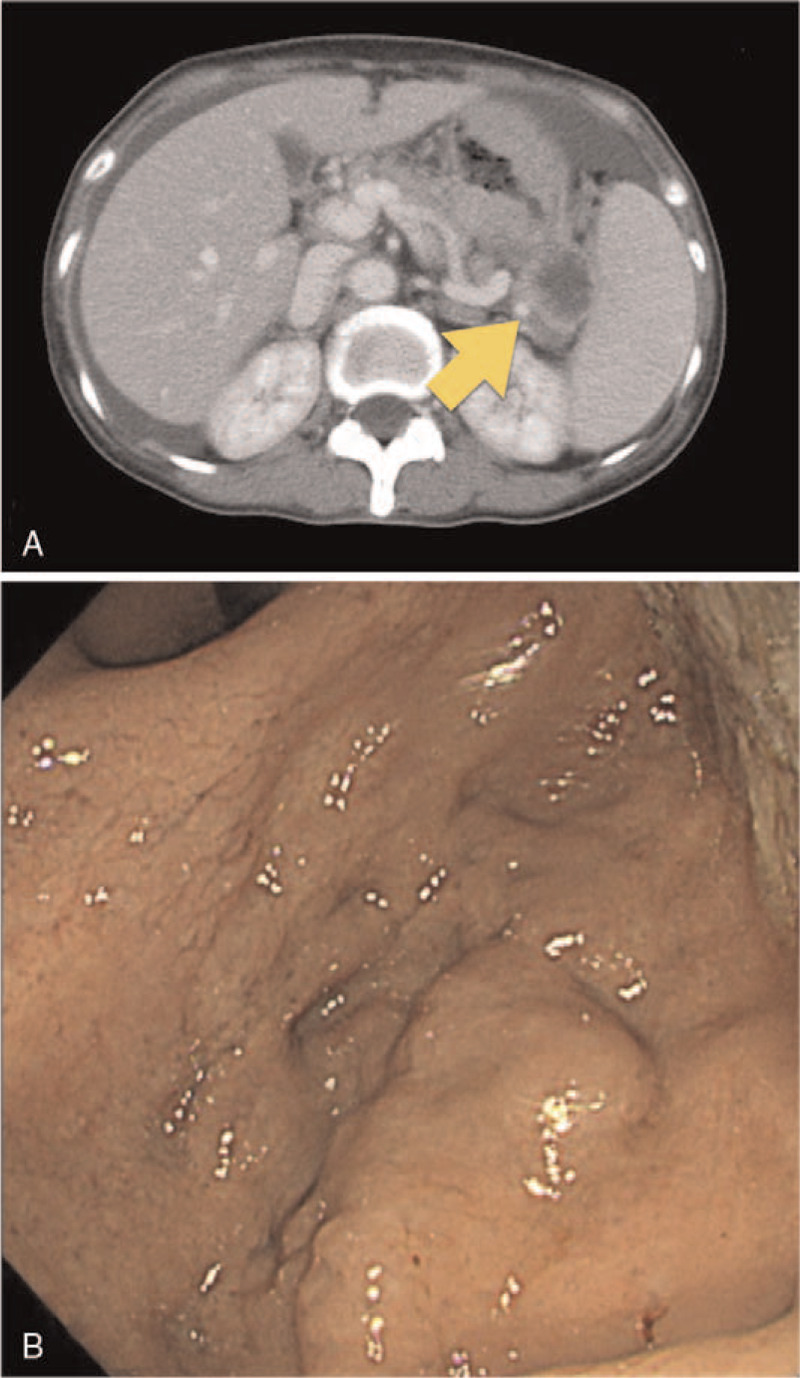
(A) Abdominal computed tomography scan showing obstruction of the splenic hilum by the tumor (arrow) in case 1. (B) Upper gastrointestinal endoscopic image showing isolated varices in the gastric fundus.
